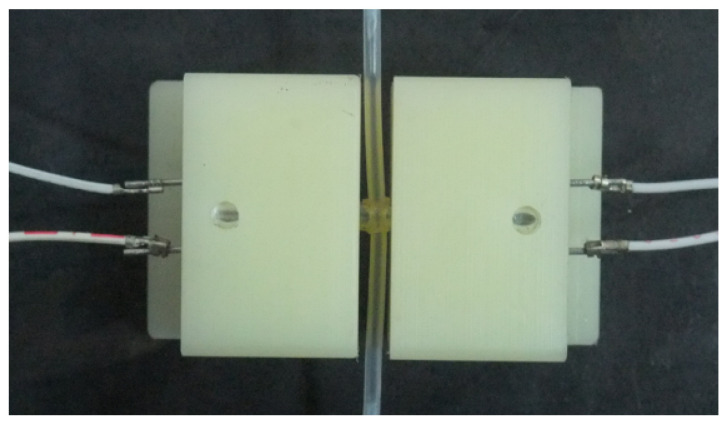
The prototype of the ultrasonic probe (top view).
